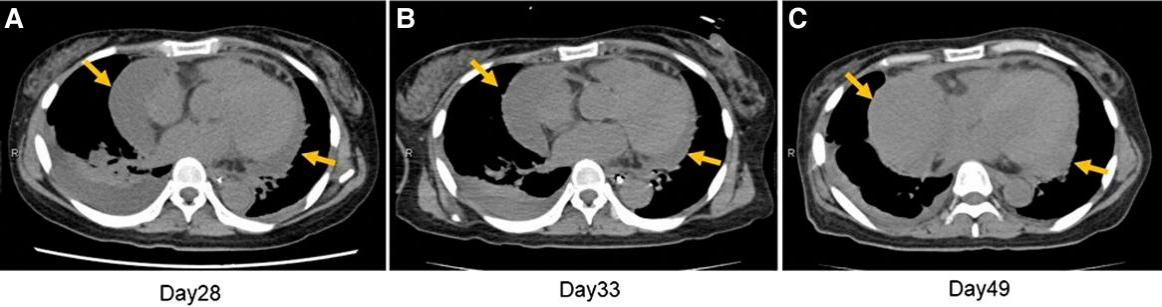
Computed tomography images after pericardiocentesis. After pericardiocentesis, computed tomography on Day 28 of admission shows residual pericardial fluid in the lateral posterior left ventricular wall and right atrial side (orange arrow) (*A*). On Day 33 of admission, computed tomography shows no significant change of residual pericardial fluid (*B*). On Day 49 of admission, computed tomography also shows a further increase in pericardial effusion (*C*).
